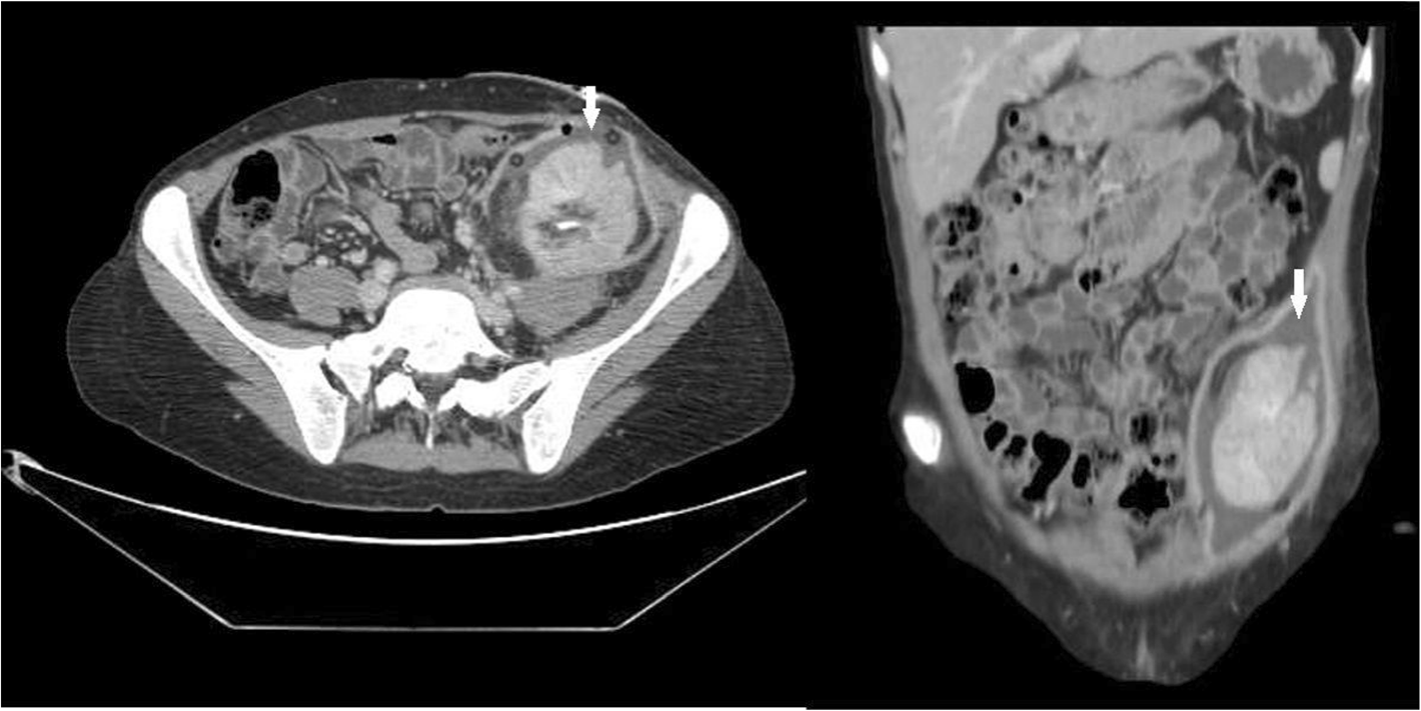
Abdomen and pelvis CT scan showing a large urine collection around the graft, in continuity with the area of the previous rupture (arrows). (left) axial reconstruction; (right) coronal reconstruction
